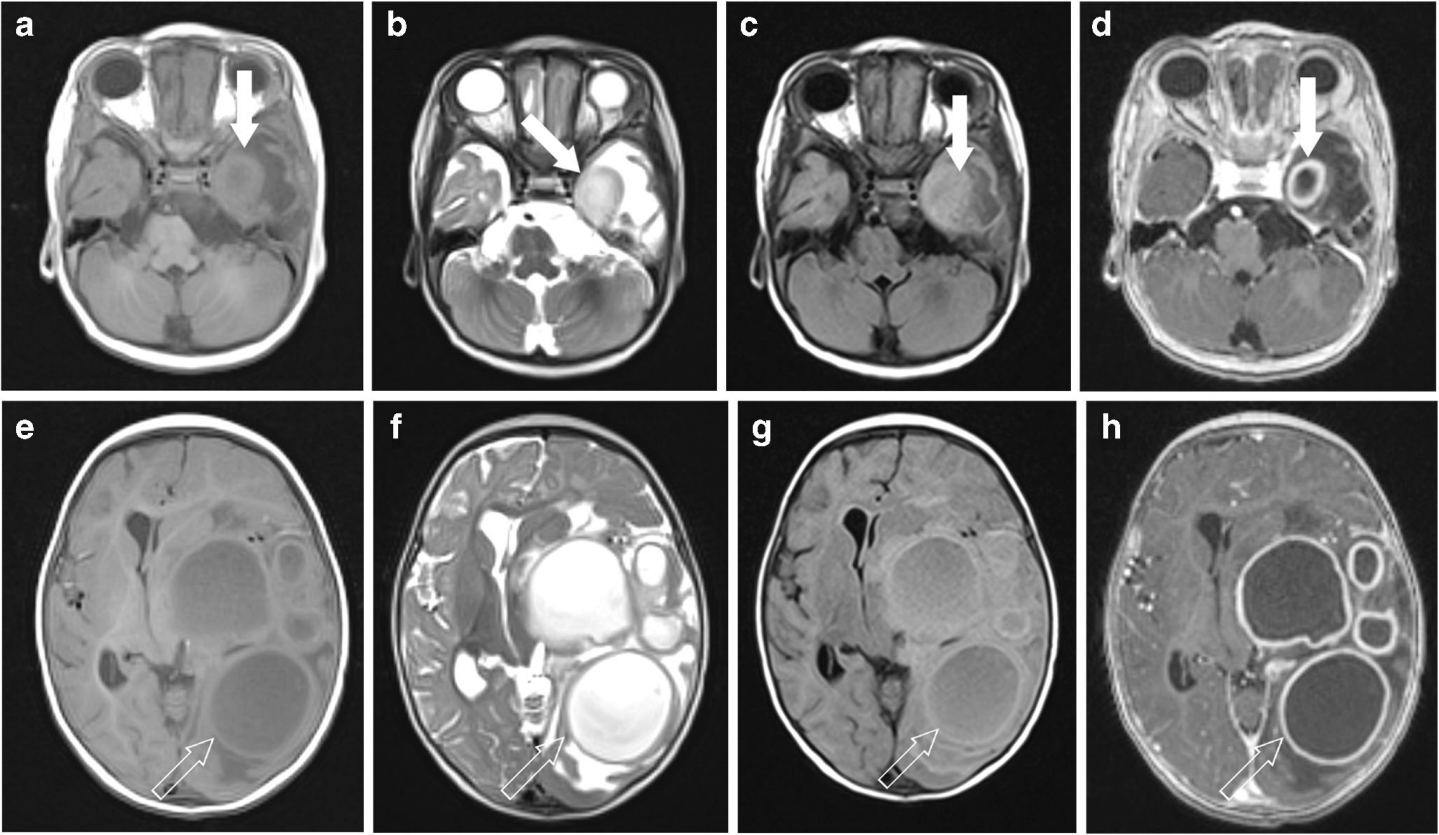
A 7-month-old boy with multifocal brain abscesses. a–d The lesion in the left temporal lobe (*solid arrows*) shows heterogeneous signal on the axial T1 (a) and T2 (b) images with mild hyperintensity and marked surrounding vasogenic edema on the axial fluid-attenuated inversion recovery (FLAIR) image (c). The post-contrast axial T1 image (d) clearly delineates a multifocal ring-enhancing lesion with thick but smooth peripheral enhancement characteristic of a cerebral abscess (likely pyogenic) in the left temporal lobe. e–h Multilocular, more cystic-appearing lesions are seen in the left frontal and parietal lobes (*open arrows*) with predominant T1 hypointensity (e) and T2 hyperintensity (f) but incomplete FLAIR signal suppression (g), associated with surrounding vasogenic edema, mass effect effacing the left lateral ventricle and rightward midline shift with mild trapping of the right lateral ventricle. The post-contrast T1 image (h) delineates multifocal ring-enhancing abscesses (likely pyogenic) in the left frontal and left parietal lobes. These findings are better delineated on post-contrast T1 images (d and h) than on pre-contrast T1 images (a and e)
